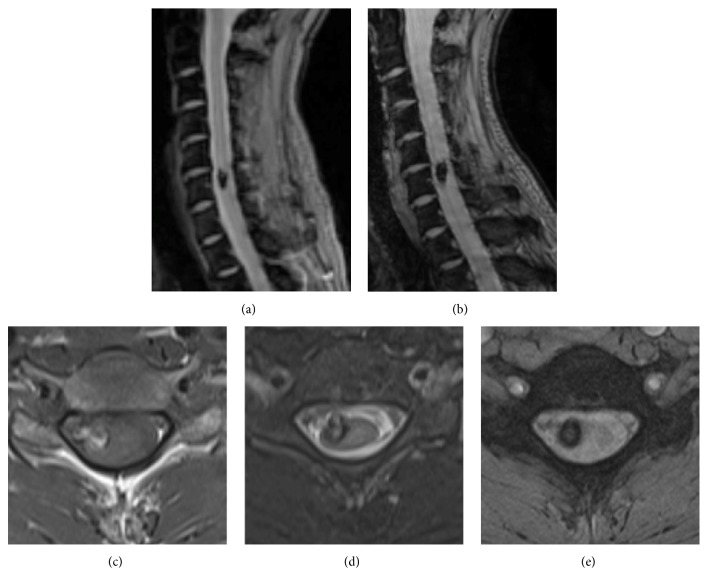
MRI images of cavernous angioma. (a) Cavernous angioma one year after SAH on T2-star sagittal image. (b) Preoperative T2-star sagittal image of cavernous angioma. The growth of the lesion is observed. (c), (d), (e) Preoperative axial image of cavernous angioma. Cavernous angioma extends laterally from the ventral side of the right half the spinal cord. Cavernous angioma is observed as a heterogenous lesion on T1 weighted image (c). Surrounding rim is seen on T2WI (d) and T2-star image (e).
